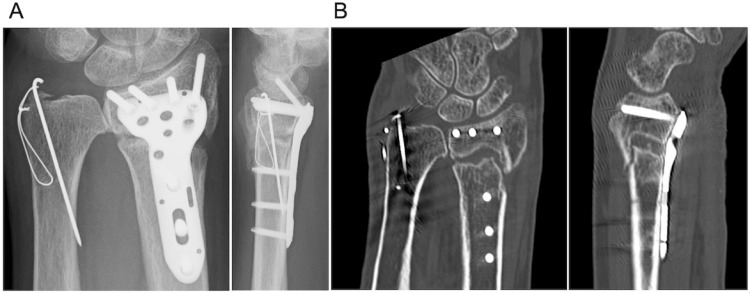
(A) Posteroanterior and lateral radiographs of the left wrist three months after the surgery. (B) Coronal image and sagittal computed tomography images three months after the surgery.
